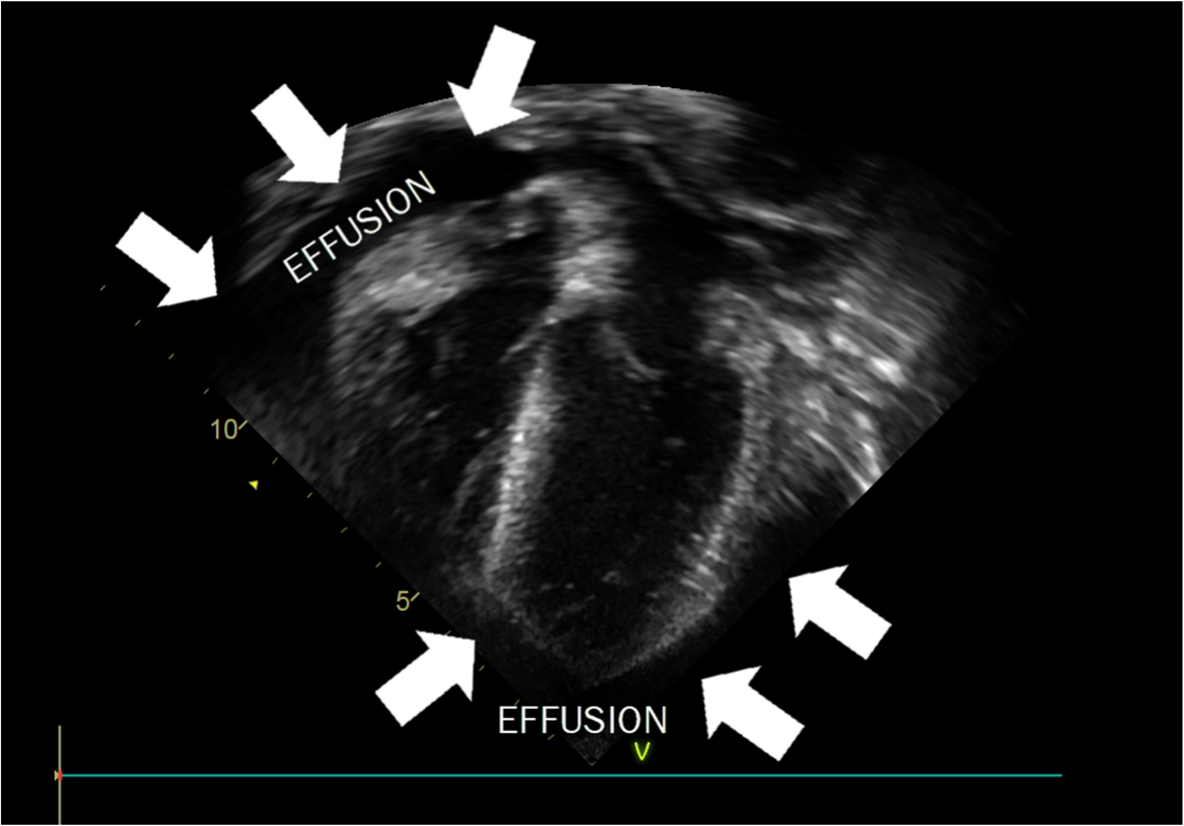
Echocardiogram showing a large circumferential pericardial effusion with diastolic collapse
